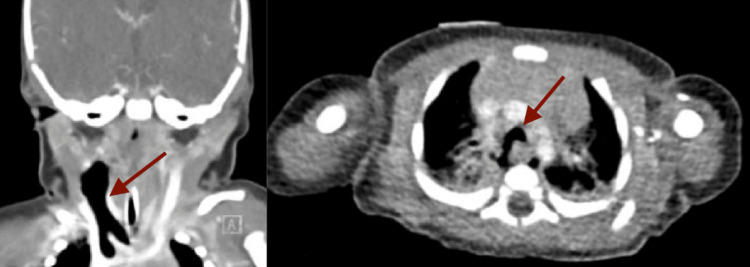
Computed tomography (CT) scan showing extensive air dissecting (arrows) through the soft tissues of the mediastinum and neck.
